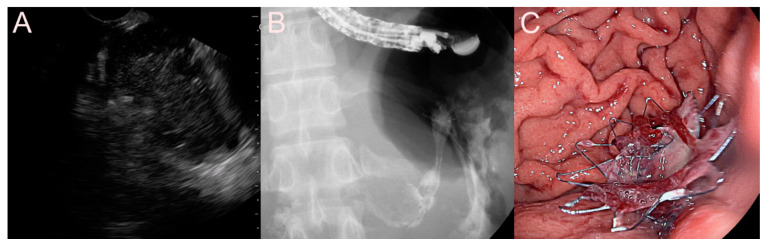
Endoscopic ultrasound-guided pancreatic fluid collection drainage using the dedicated bi-flanged, fully covered self-expandable metal stent (FCSEMS) (BONA-AL Stent; Standard Sci Tech Inc., Seoul, Korea) for a walled-off necrosis (WON). (A) Endosonographic image showing a WON with heterogeneous necrotic debris. (B) Fluoroscopic image showing the FCSEMS placed between the stomach and the WON. (C) Endoscopic image showing the drainage of pus-like materials and large amounts of sludge through the FCSEMS.
